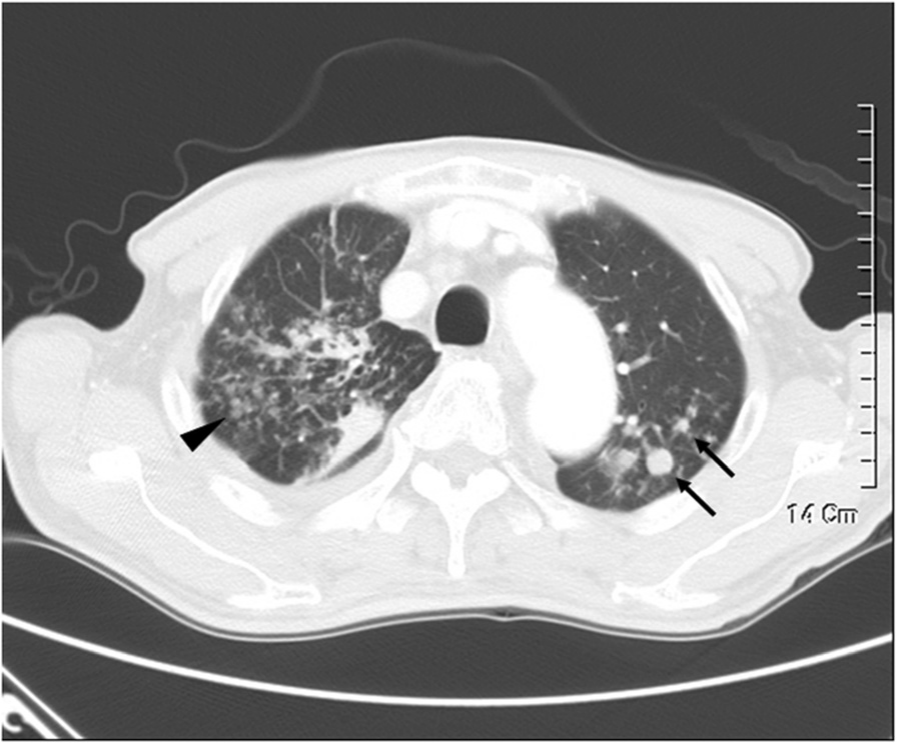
Thin section CT of a 72–year-old male with pulmonary tuberculosis demonstrating nodules (arrows) and tree-in-bud pattern (arrowhead); loculated pleural effusion at right lower lung zones.
